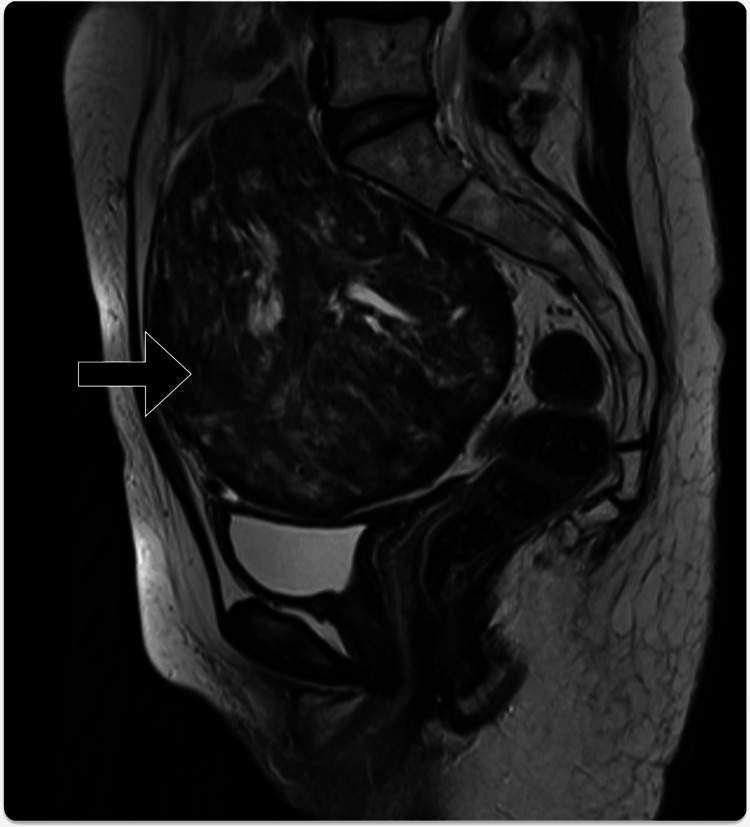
T2-weighted MRI showing a 21×12×15 cm tumor in the pelvic cavity
